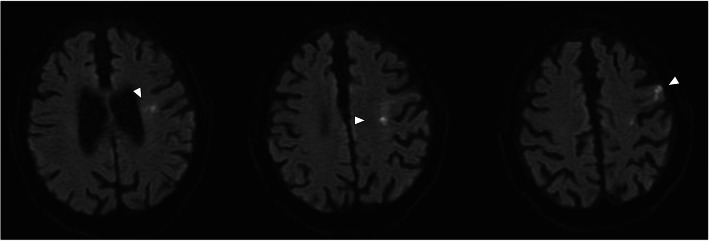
Diffusion-weighted MRI of brain. Axial diffusion-weighted MRI showing a high-signal-intensity area in the patient’s left brain, indicating acute cerebral infarction on the left cortex of the frontal lobe and radiate crown
